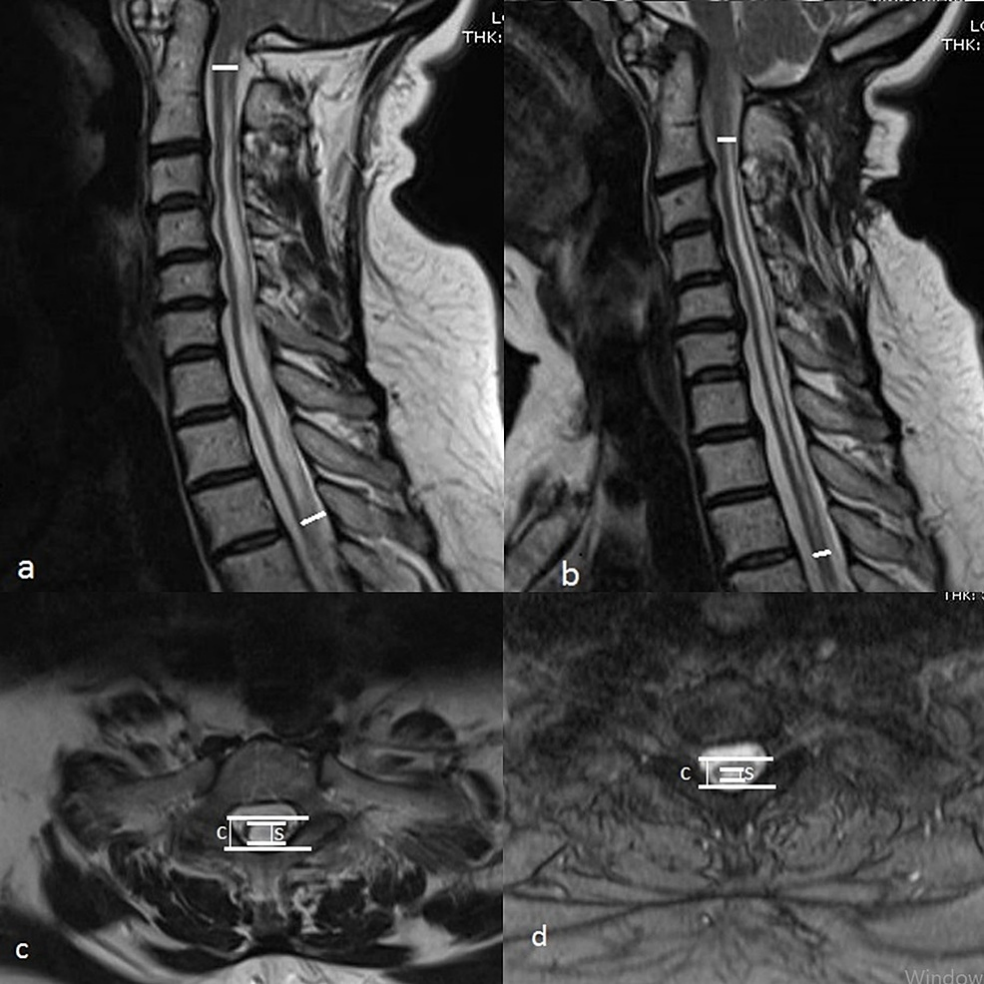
The pre- and postoperative MRIs of a 50-year-old female patient with CM1. The pre- and postoperative MRIs of a 50-year-old female patient with CM1: (a) Preoperative MRI demonstrated a syrinx extended from the C2 to T3 vertebrae level (b) The sixth postoperative month MRI showed no significant regression of the syrinx’ length; however, the patient’s presenting symptoms improved related to the regression in the S/C ratio (c) Preoperative axial MRI showed S/C ratio (d) Postoperative sixth MRI showed a regressed S/C ratio. S/C ratio: syrinx/cord ratio; CM1: Chiari malformation type I
